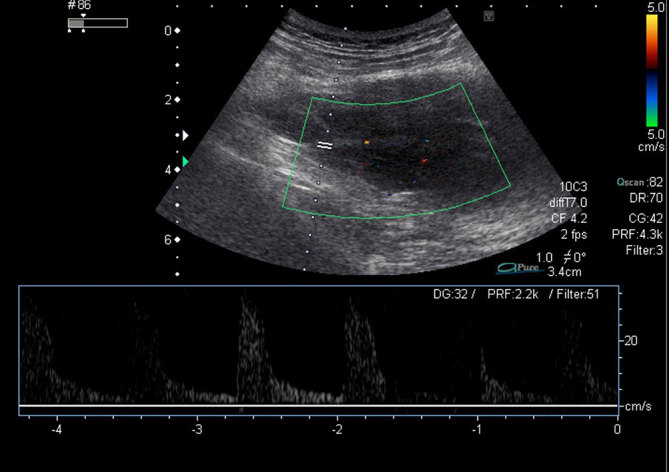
Doppler ultrasound showing a hypoechoic solid intramuscular mass affecting the right gluteus maximus and demonstrating arterial flow within the mass.
